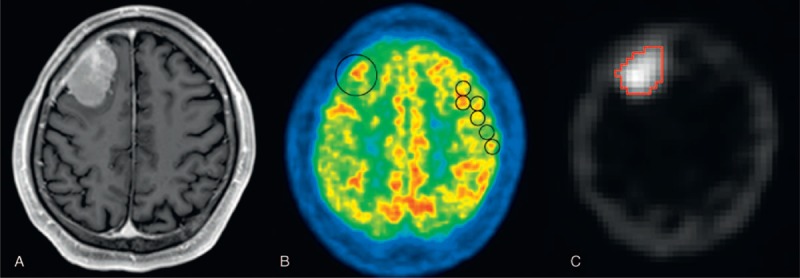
Contrast-enhanced MRI (A), FDG-PET (B), and Tl-SPECT (C) images of a 53-year-old patient with WHO grade II meningioma, atypical type. The FDG-PET image (B) and Tl-SPECT image (C) show high uptake in the tumor at the right frontal region. (B)The circles show examples of drawn ROI. (C)The tumor is selected with a threshold algorithm. The SUVmax, SUVRmax, TURmean, and TURmax were 8.69, 0.89, 11.92, and 16.57. FDG = fluorine-18 fluorodeoxyglucose, PET = positron emission tomography, ROI = region of interest, SPECT = single photon emission computed tomography, SUV = standardized uptake value, SUVmax = the maximum value of SUV, SUVRmax = the maximum SUV ratio, Tl = thallium-201, TURmax = the maximum Tl uptake ratio, TURmean = the mean Tl uptake ratio.
